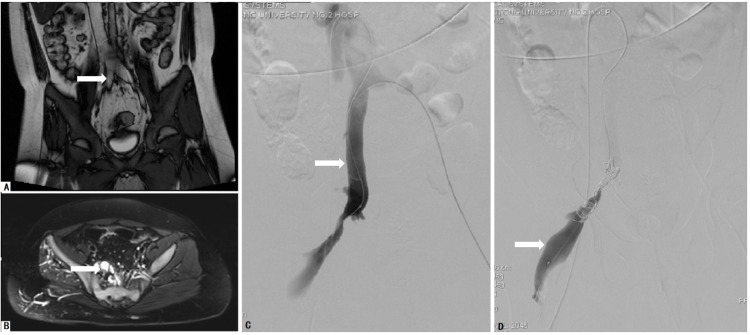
Images of a 12-year-old boy with KTS with gross anorectal bleeding who underwent trans-femoral vein radiologically interventional IIV embolization and sclerotherapy (FRIIVES) A: MRI revealed an aberrant and dilated IIV (arrow). B: The malformed IIV (arrow) showed a higher signal than the contralateral normal vein on the T2-weighted axial MRI scan, which implied IIV reflux. C and D: The malformed IIV (arrow) was managed with coils, ethanol, and foamed polidocanol for ablating bleeding IIV: internal iliac vein; KTS: Klippel-Trénaunay syndrome; MRI: magnetic resonance imaging
